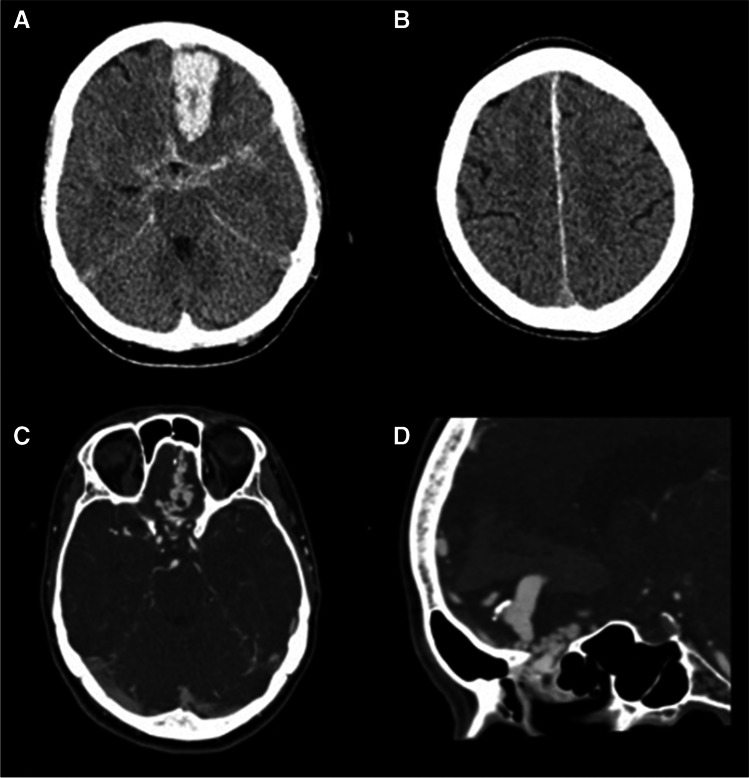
Selected case (patient case 5 from Table 1). A Sixty-three female presenting with sudden onset headache, nausea, and vomiting. Non-contrast CT head study demonstrated left frontal acute intraparenchymal hemorrhage with subarachnoid hemorrhage and B left parafalcine subdural hemorrhage. C, D Subsequent CT angiography (CTA) highlighted a network of several serpiginous underlying draining veins within the anterior cranial fossa
